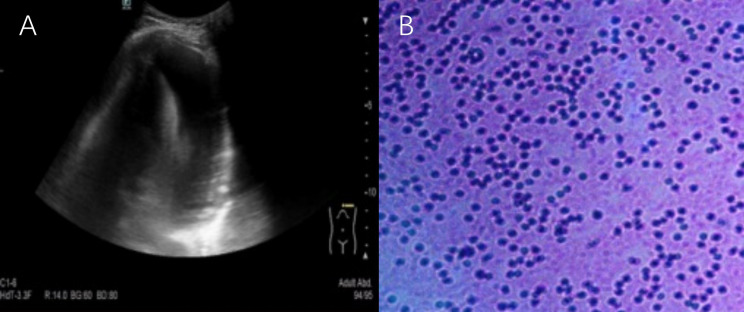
Characteristics of pericardial effusion. (A) Ultrasound shows a large amount of pericardial effusion. (B) Cytological analysis of the pericardial fluid reveals predominantly inflammatory cells with no evidence of malignancy.
